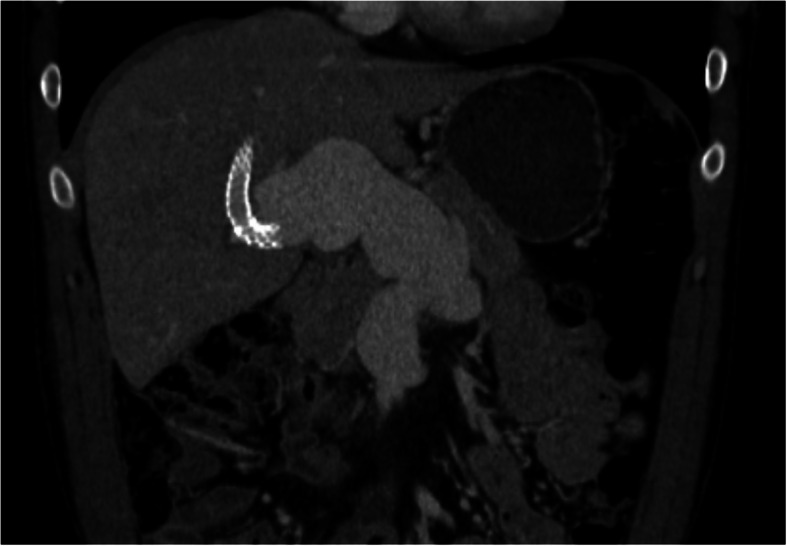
Coronal contrast-enhanced CT showing the widely patent portomesenteric aneurysm 1-month post-intervention. The right hepatic vein-to-right posterior portal vein TIPS is also patent
